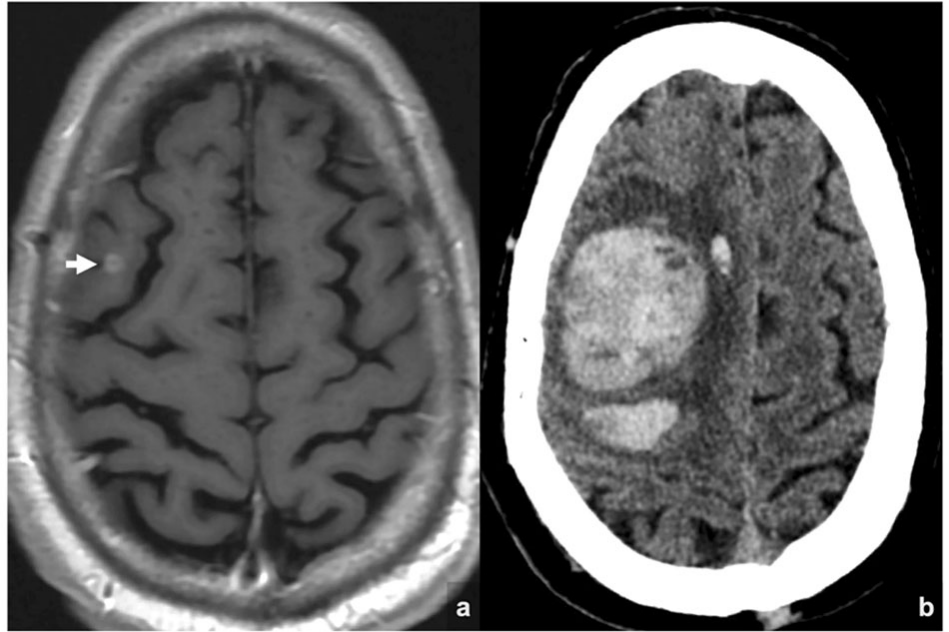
Hemorrhagic transformation of metastasis from melanoma treated with Nivolumab–Ipilimumab and WBRT. Brain MRI performed 51 days prior to the initiation of treatment (a) showed a right frontal metastasis. Fifty-two days after starting treatment, the patient presented to the emergency department with sudden-onset left hemiparesis. A cranial CT scan (b) performed the same day revealed a right frontal intraparenchymal hemorrhage consistent with hemorrhagic transformation of the known brain metastasis
